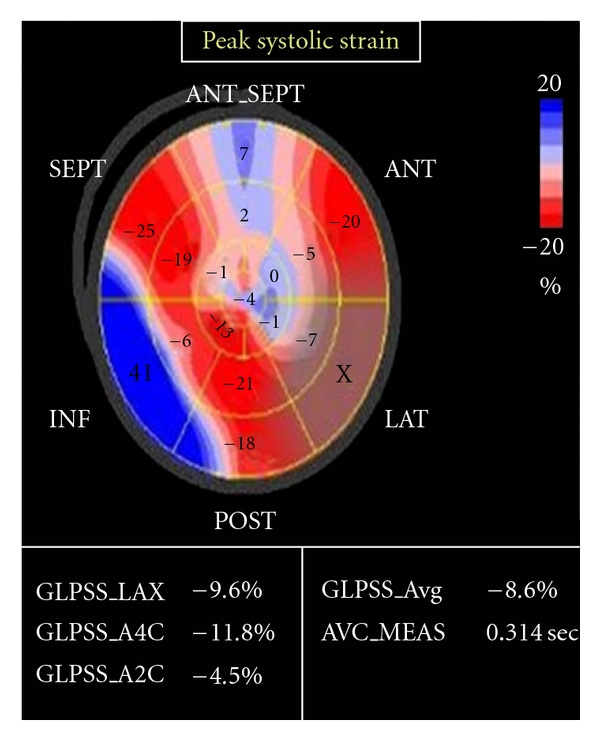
2D strain echocardiography in patient with doxorubicin-induced cardiotoxicity.
